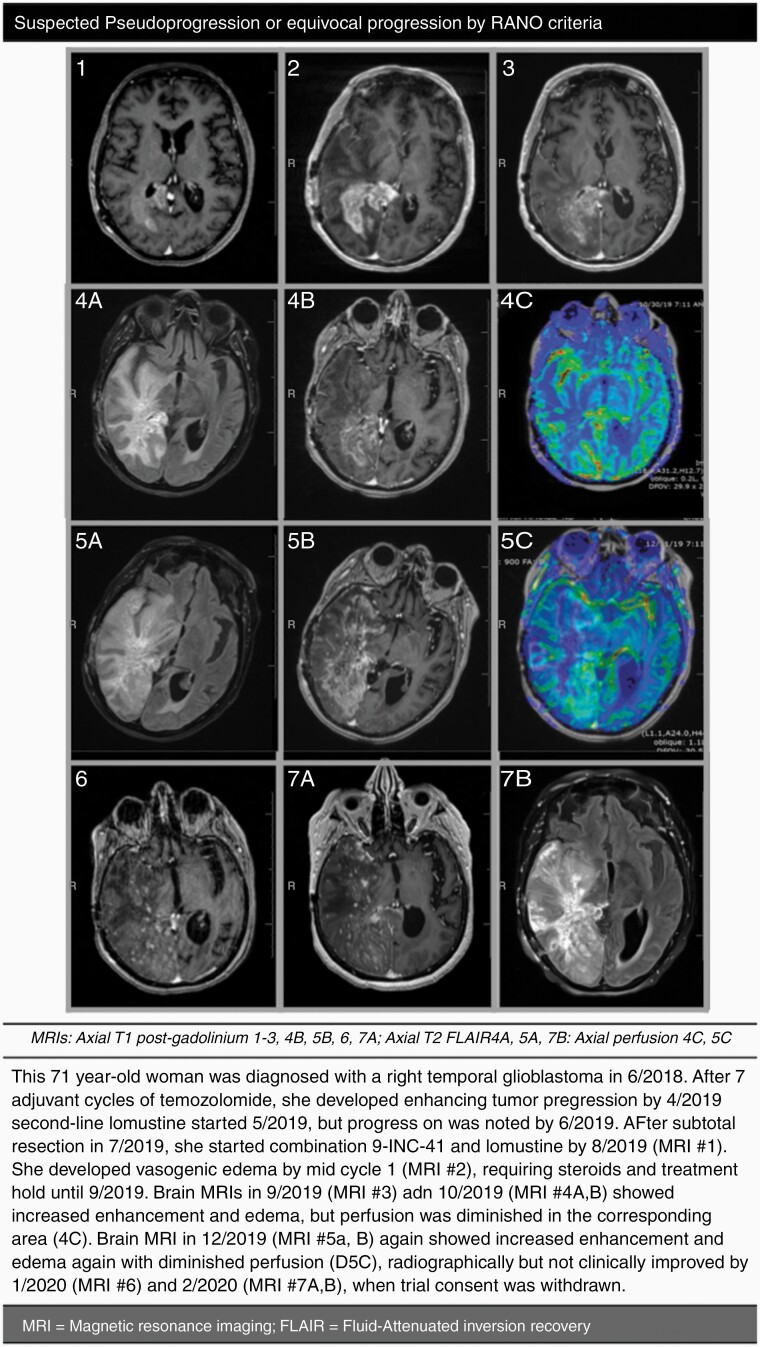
Suspected pseudo-progression or equivocal progression by RANO criteria. This 71-year-old woman was diagnosed with a right temporal glioblastoma in 6/2018. After 7 adjuvant cycles of temozolomide, she developed enhancing tumor progression by 4/2019. Second-line lomustine started 5/2019, but progression was noted by 6/2019. After subtotal resection in 7/2019, she started combination 9-ING-41 and lomustine by 8/2019 (MRI #1). She developed vasogenic edema by mid cycle 1 (MRI #2), requiring steroids and treatment hold until 9/2019. Brain MRIs in 9/2019 (MRI #3) and 10/2019 (MRI #4A,B) showed increased enhancement and edema, but perfusion was diminished in the corresponding area (4C). Brain MRI in 12/2019 (MRI #5A,B) again showed increased enhancement and edema again with diminished perfusion (5C), radiographically but not clinically improved by 1/2020 (MRI #6) and 2/2020 (MRI #7A,B), when trial consent was withdrawn.
